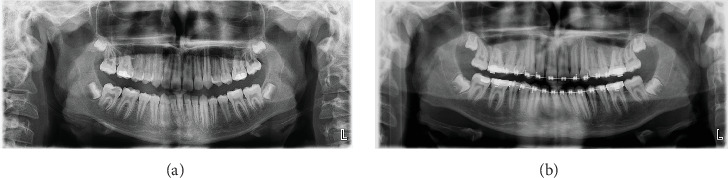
Panoramic X-rays (a) before and (b) 2 years after the onset of treatment.
